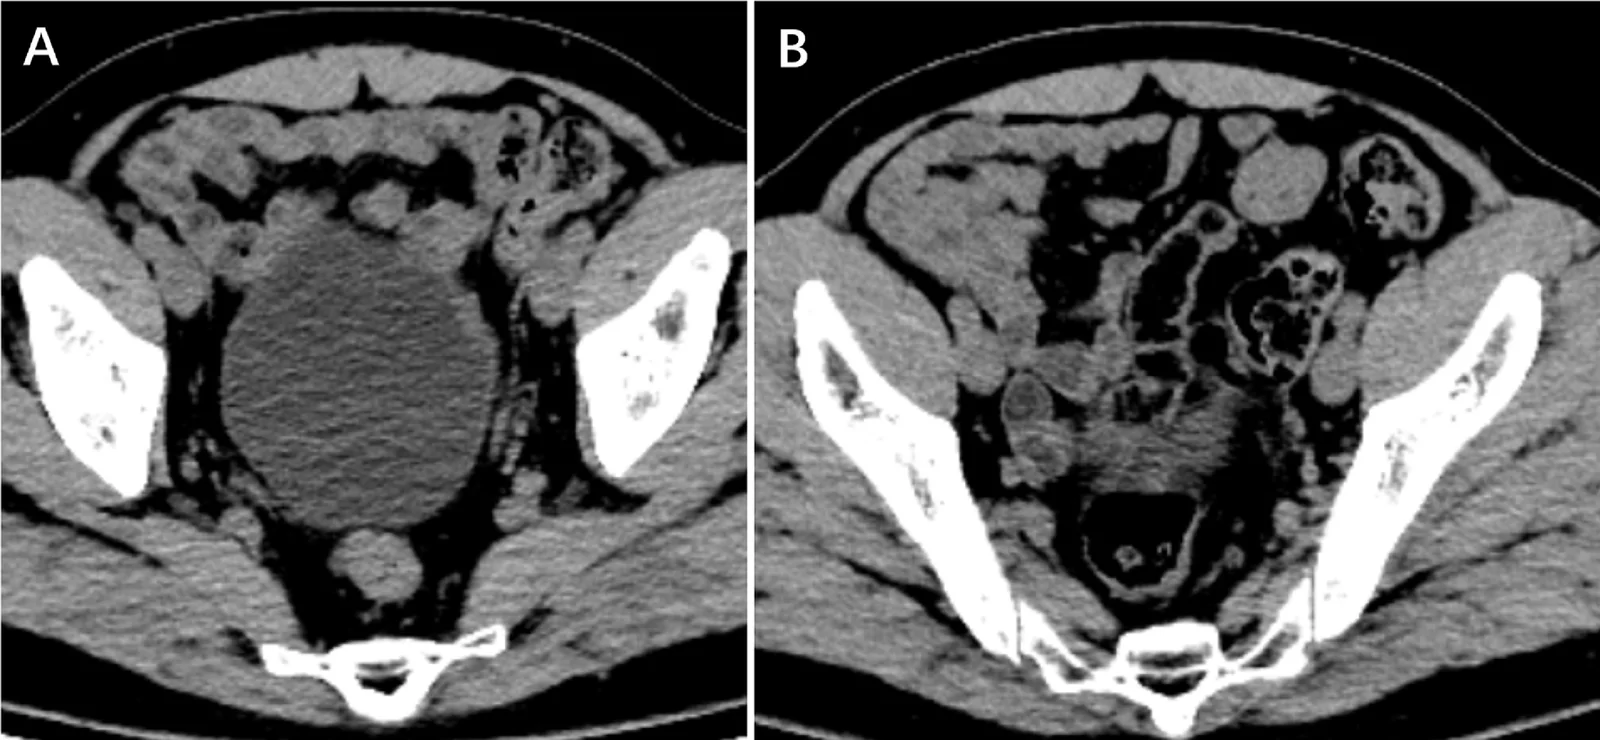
Follow-up CT after non-operative management. Follow-up CT obtained 3 months later at a comparable level demonstrates resolution of the extraluminal air and substantial improvement in the appendiceal and adjacent sigmoid inflammatory changes.
